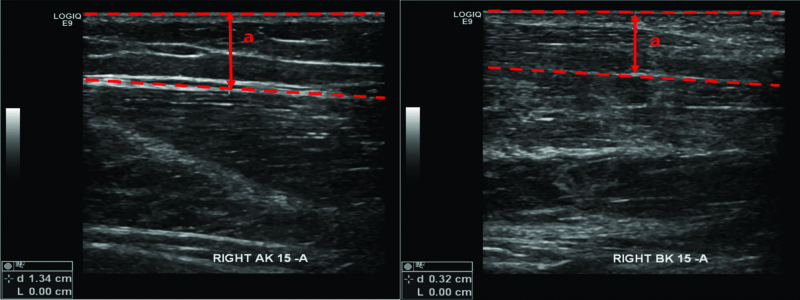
Ultrasonographic measurement of ΔCSA in the lower extremity (a: distance between skin and the fascia of the muscle). AK = above the knee, BK = below the knee, ΔCSA = cross-sectional area.
